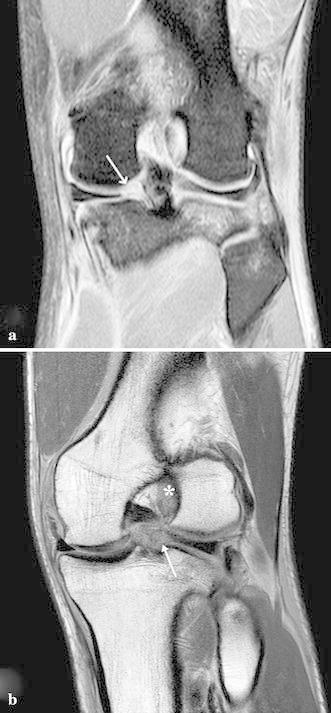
a Preoperative coronal TSE-fat saturated MR image shows the ACL tear and an area of heterogeneous intrameniscal signal intensity of both meniscal roots with a void at the posterior attachment site of both menisci (*arrow*). b Preoperative coronal oblique 30° MR image. The *arrow* shows absence of tibial insertion of posterior lateral root. The *asterisk* shows hyperintensity signal at femoral ACL insertion
